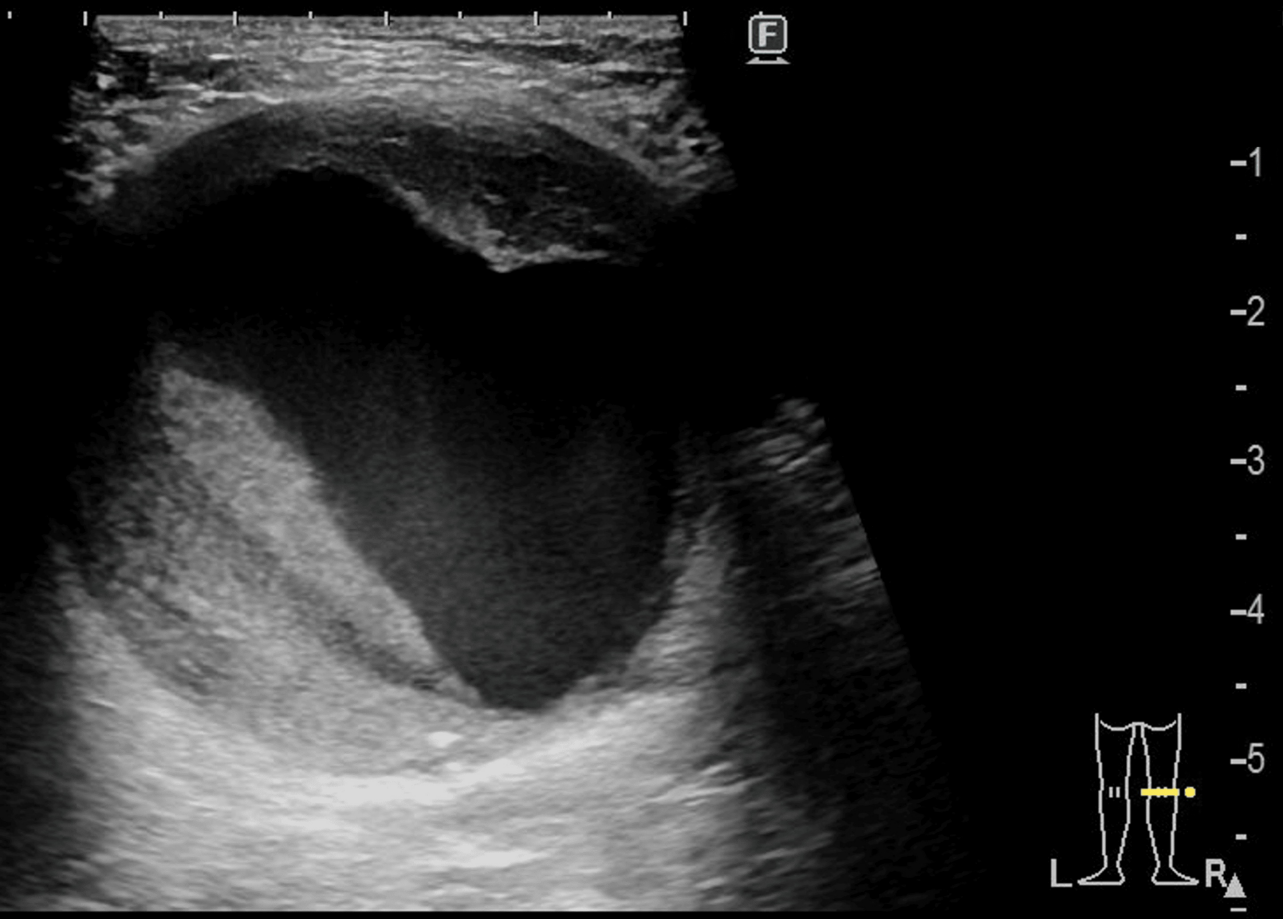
A right popliteal artery aneurysm was an incidental finding on duplex ultrasonography.
